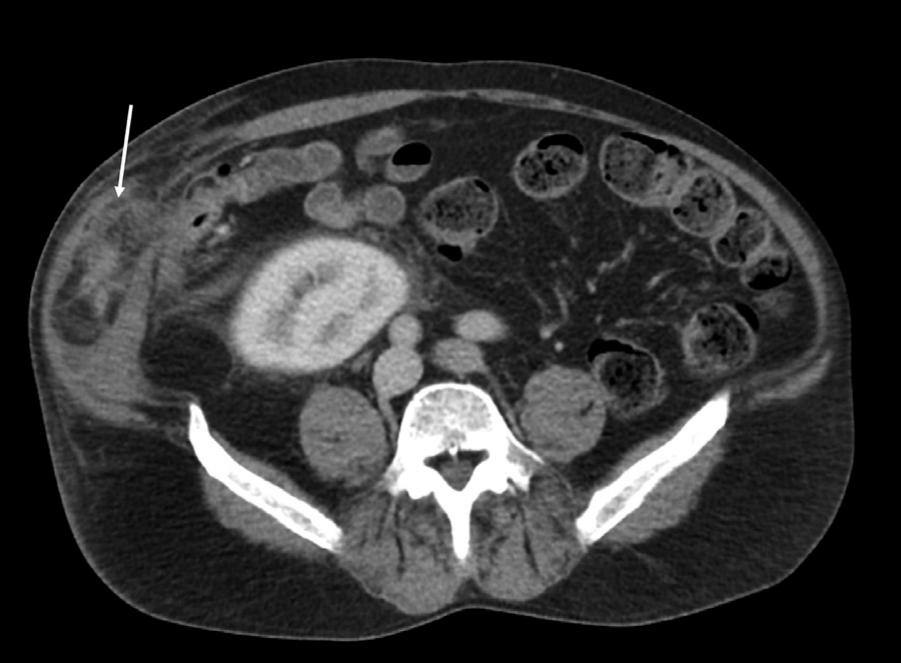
CT abdomen and pelvis with intravenous contrast administration, axial view demonstrates a wide necked incisional hernia in the right lower quadrant containing a tubular structure (white arrow) with associated diffuse edema in the hernial sac. Of note: The image also includes, transplanted kidney in the right Iliac Fossa.
